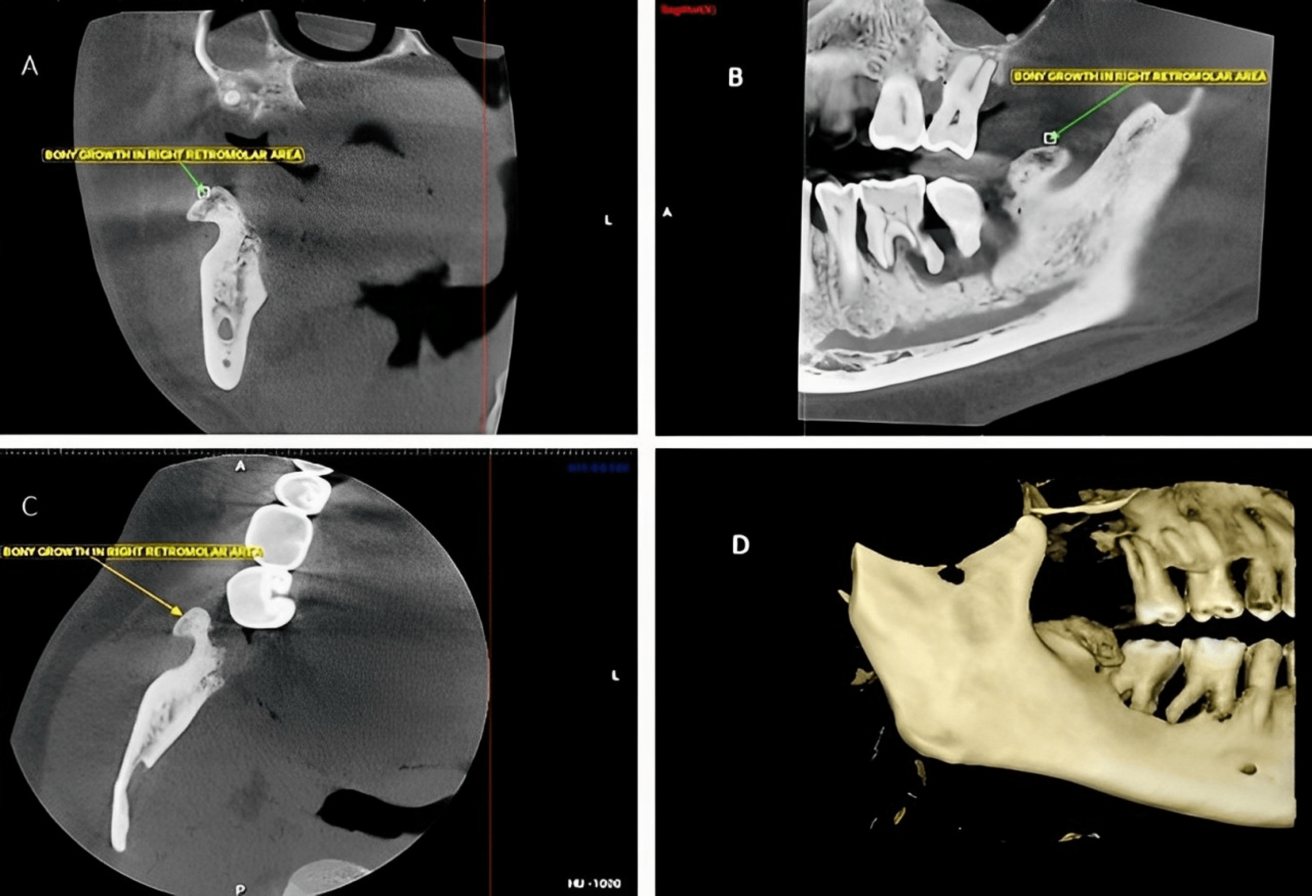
CBCT findings of the bony growth seen in the right retromolar region A: coronal view of the bony growth in the retromolar region; B: sagittal view of the bony growth in the retromolar region; C: axial view of the bony growth in the retromolar region; D: 3D reconstruction of the bony growth in the retromolar region CBCT: cone beam computed tomography
